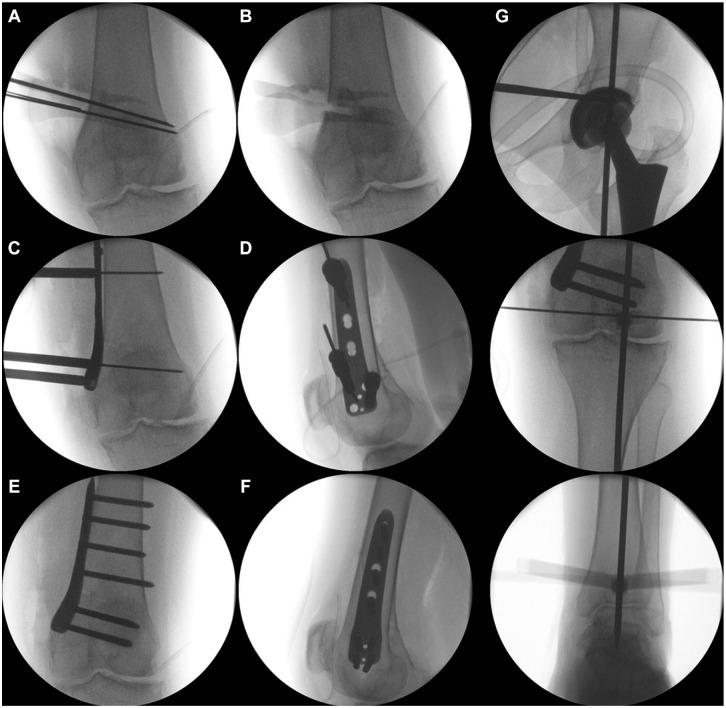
Intraoperative radiographs of the distal femoral osteotomy: positioning of the K-wires. (A) The hinge is defined as the intersection of the shadow of the posterior lateral condyle and the lateral cortex. (B) Removal of the bone wedge. (C, D) Plate position. (E, F) Radiographs of the completed distal femoral osteotomy. (G) Intraoperative control of the alignment using the alignment rod.
